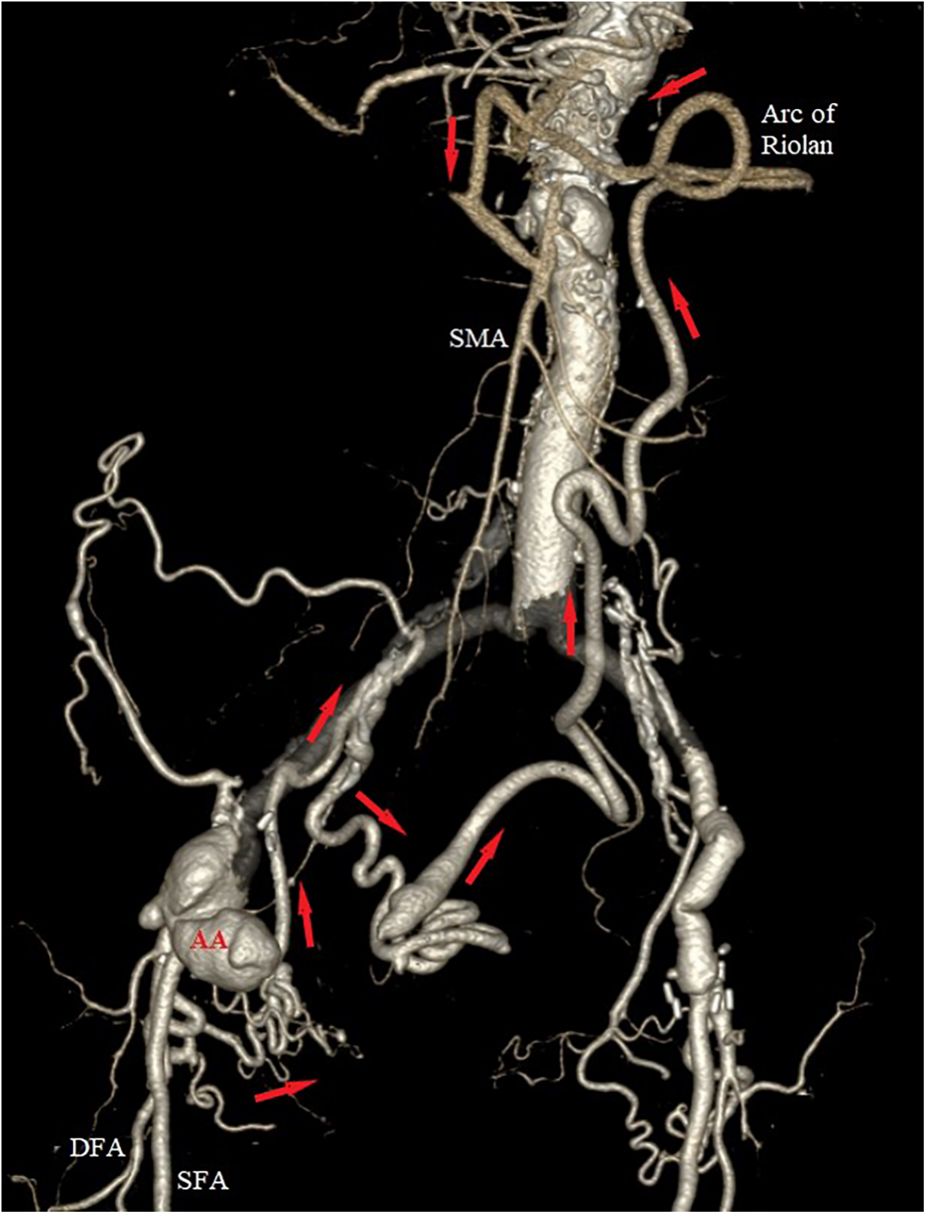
Preoperative 3D volume rendering of the computed tomography angiography (CTA), showing the anastomotic aneurysm (AA) of the right groin, with retrograde perfusion of the superior mesenteric artery (SMA) via a highly developed collateral network (red arrows) from the right deep femoral artery (DFA). Notice the ostial occlusion of the right hypogastric, the inferior and superior mesenteric arteries, and the well-developed arc of Riolan.
